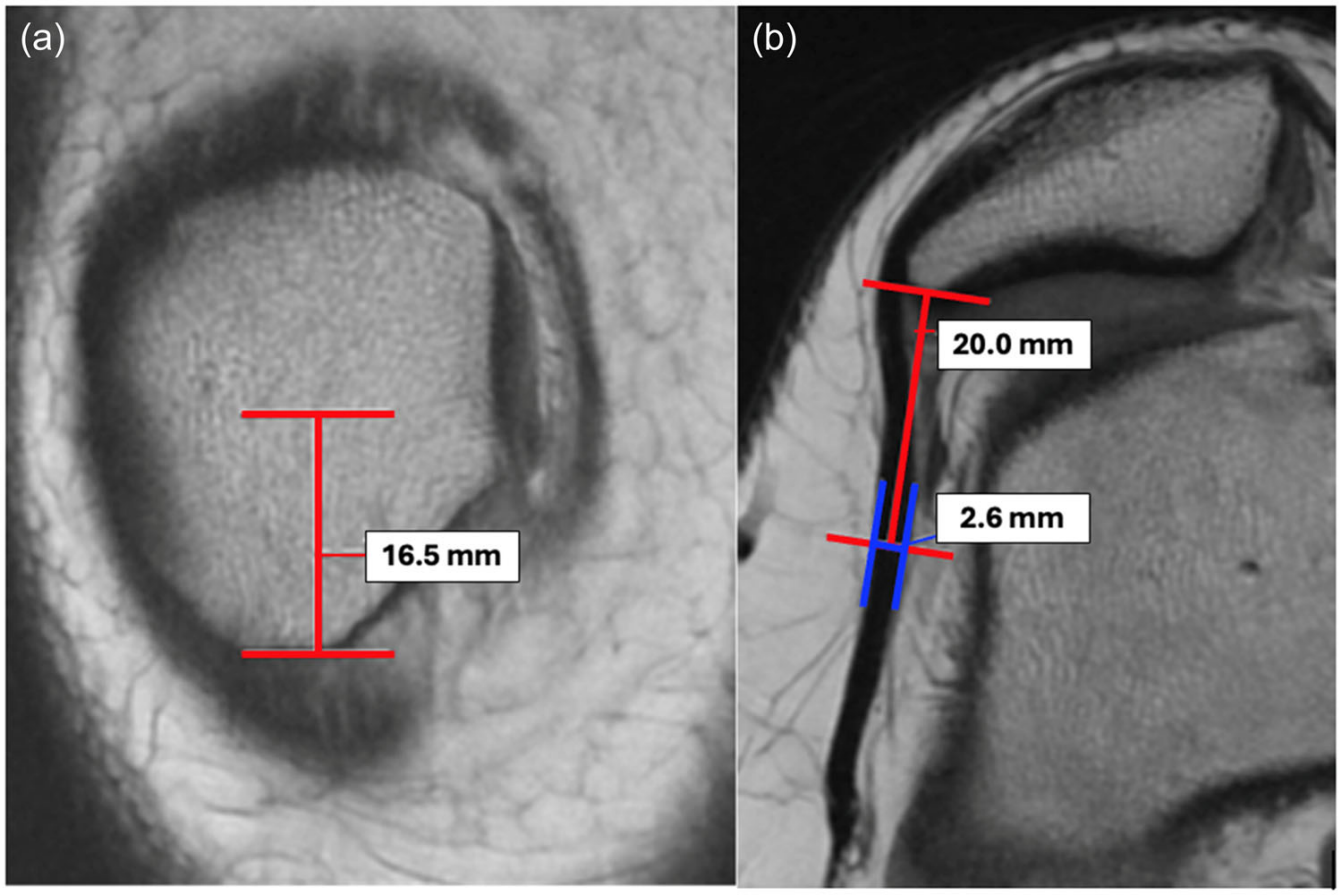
LPR Measurement on MRI. (a) Coronal MRI slice with longest height of patella identified, distance measured and divided by half to identify the midpoint (ex: 16.5 mm). (b) Midpoint from coronal view used to identify slice on axial view, 20 mm measured from lateral edge of patella to LPR, at this point LPR thickness is measured (ex: 2.6 mm). LPR, lateral patellar retinaculum; MRI, magnetic resonance imaging.
